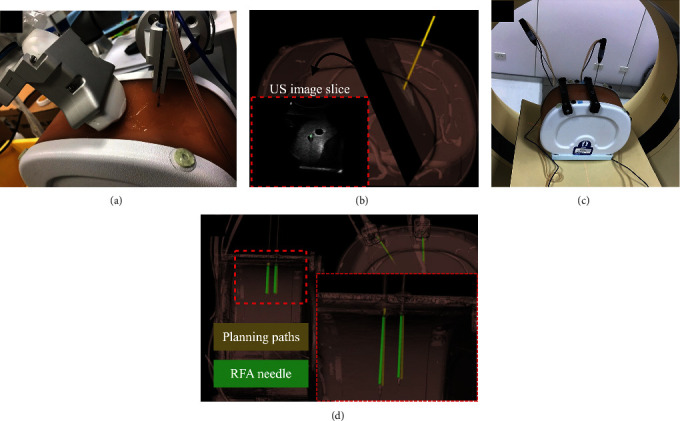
The RFA insertion with US image-guided experiment. (a) US image-guided RFA insertion. (b) The needle was visualized by 3D reconstruction on the CT image with US image slice (as shown in bottom left). The green point shows the needle position. (c) The CT scan after insertion. (d) The reconstructed planning path and the RFA needle from the CT image.
